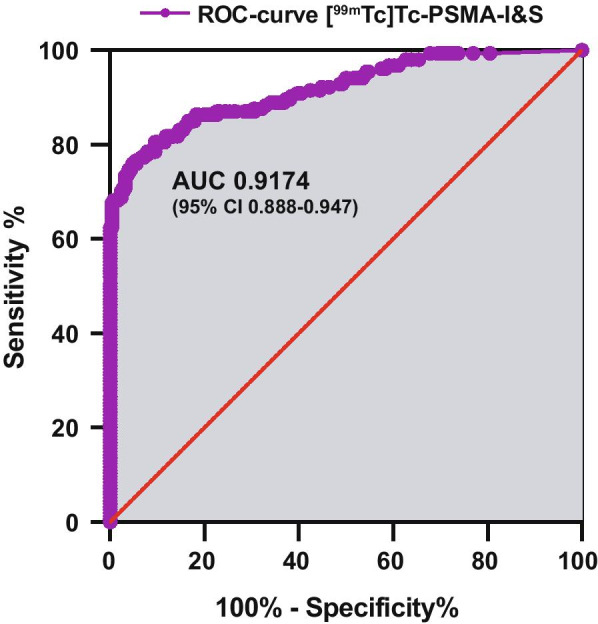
ROC analyses revealed an AUC of 0.9174 (95% CI 0.89–0.95) for [^99m^Tc]Tc-PSMA-I&T (154 samples with tumor, 252 samples without tumor)
